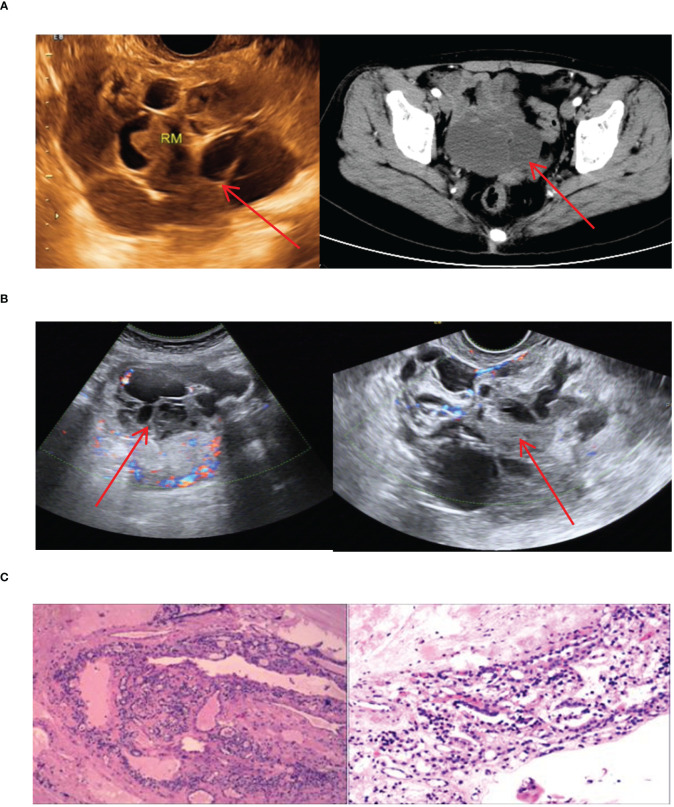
Imaging manifestations and pathological findings of ovarian ETG (A, B): Both ultrasound and CT images showed a cystic or cystic-solid mass in the adnexal area, which had poor confluency and multiple cystic cavities of different sizes and irregular shapes. (C): The postoperative pathology showed the nodular goiter in the ovary. Both ultrasound and CT showed a cystic or solid cystic mass in the adnexal area with poor fusion and multiple irregularly shaped sacs of different sizes.
